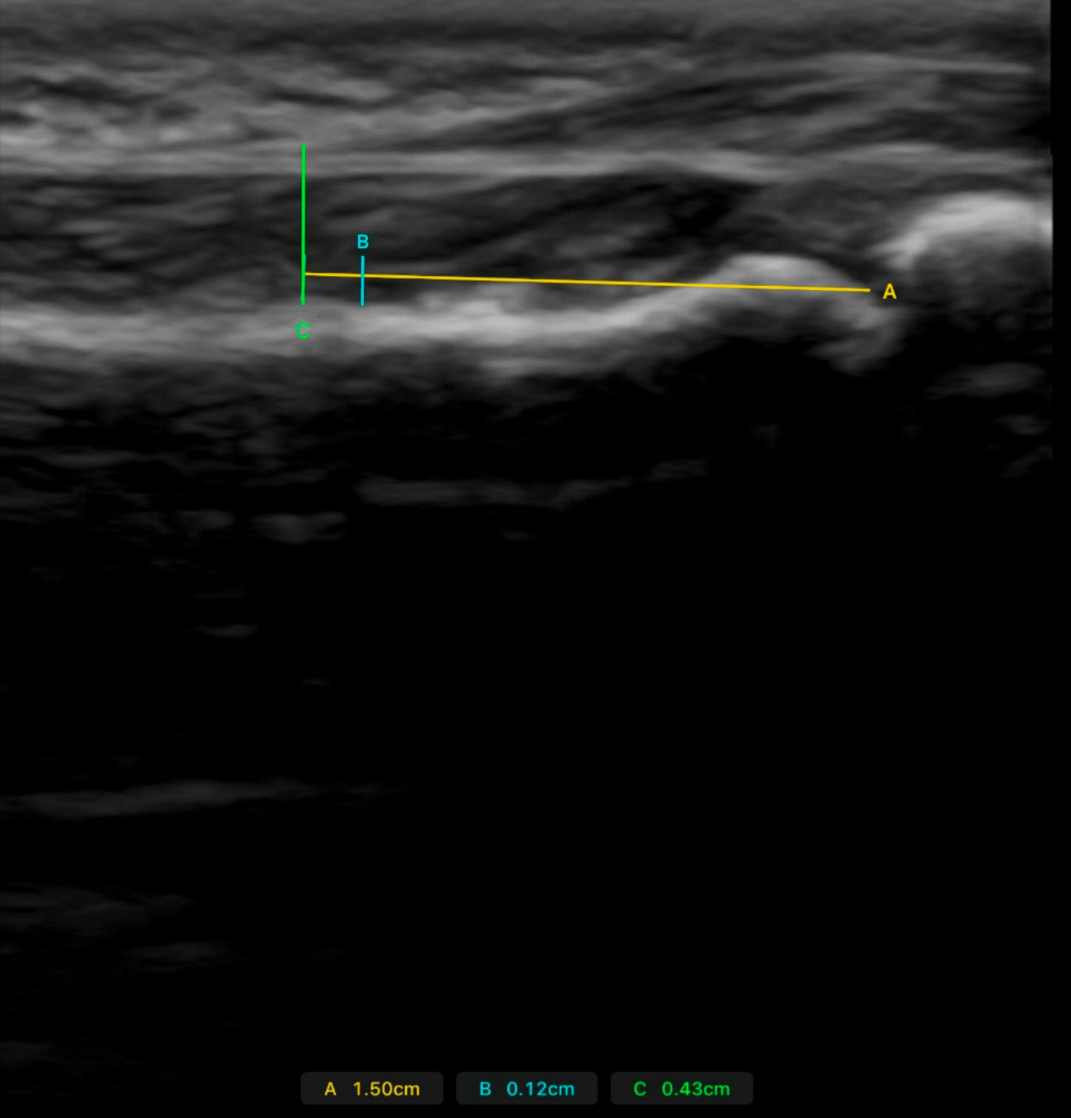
A longitudinal ultrasound view of the finger flexor tendon. Distance A (yellow line) represents the distance to the midpoint of the proximal phalanx, distance B (blue line) represents the distance between the tendon and the bone, and distance C (green line) represents the distance from the superficial border of the tendon sheath to the bone.
